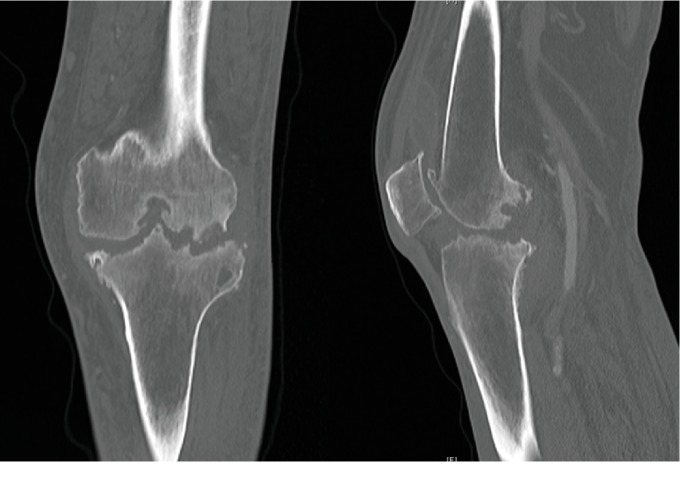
Left knee coronal and sagittal computed tomography (CT) images demonstrating bony destruction of the distal femur and proximal tibia.
